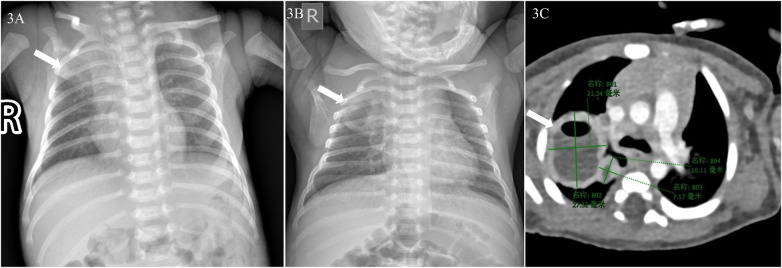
Multimodal imaging findings of lung abscess secondary to neonatal varicella. (A) Chest x-ray on illness day 6 (hospital day 4) showing right upper lobe consolidation (arrow). (B) Chest x-ray on illness day 9 (hospital day 6) demonstrating progression with suspected abscess formation (arrow). Characterization phase: (C) Coronal multiplanar reconstruction (MPR) CT image showing a right upper lobe abscess measuring 21 × 27 × 23 mm (arrow).
